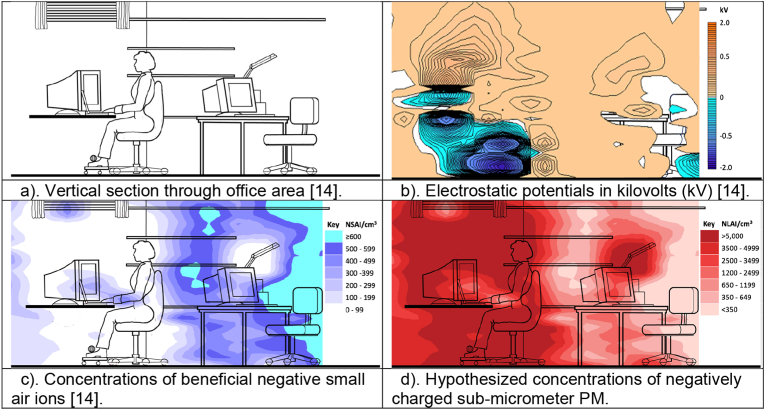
Cross-section through radiographers' suite in Bergen, Norway.
